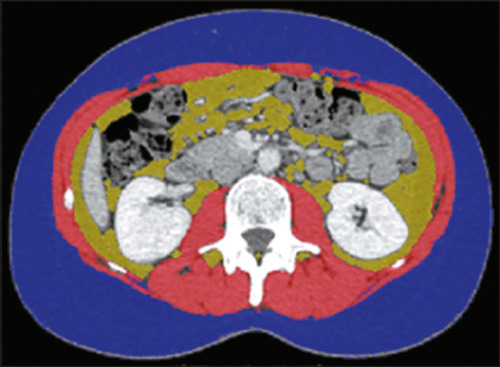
Example of a CT image at the level of the third lumbar vertebra
